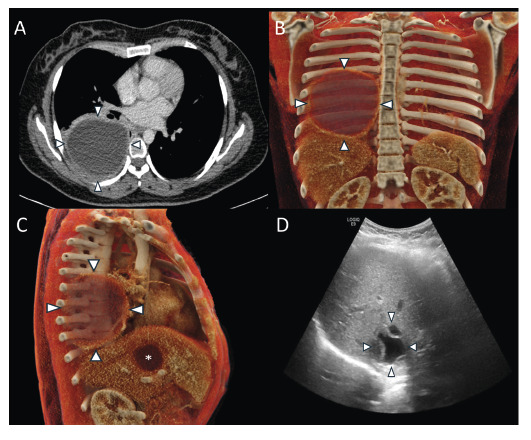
(A) Axial contrast-enhanced chest CT shows a large, well-defined cystic lesion in the right lower lobe of the lung (white arrowheads). (B,C) Cinematic volume-rendered reconstructions redemonstrate the lung (white arrowheads) and liver (asterisk) cystic lesions. (D) Abdominal ultrasound showing a well-defined cystic lesion in the right hepatic lobe containing a detached, irregular laminated membrane floating within its contents, consistent with the “water lily sign.”
